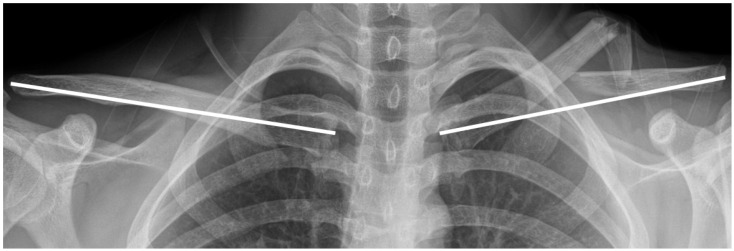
Clavicle length was measured from the most lateral to the most medial point of the anteroposterior radiograph. The length of the fractured clavicle was compared with that of the uninjured contralateral side for the calculation of clavicle shortening (both white lines).
